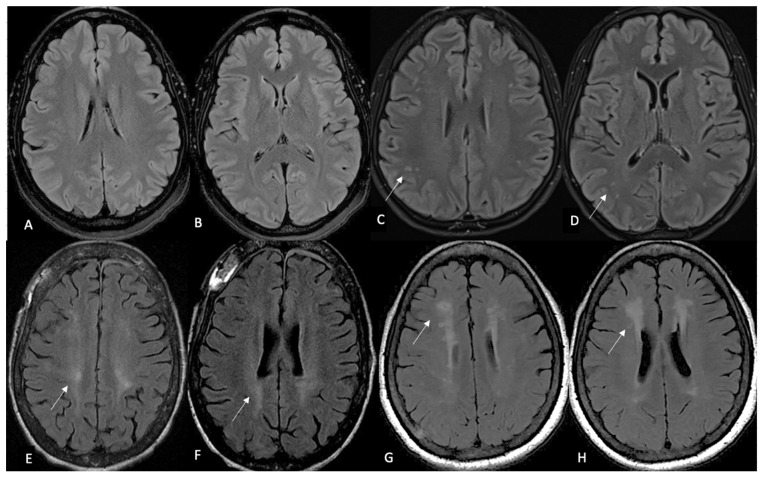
Multiple axial (Ax) T2-FLAIR MR images (A–H) depicting Fazekas scores 0–3. (A,B): demonstrate Fazekas 0, with no evidence of WMD; (C,D): show rare focal WM hyperintensities (white arrows), Fazekas 1; (E,F): show beginning of confluence of WM hyperintensities (white arrows), Fazekas 2; (G,H): show diffuse involvement of periventricular WM (white arrows), Fazekas 3.
